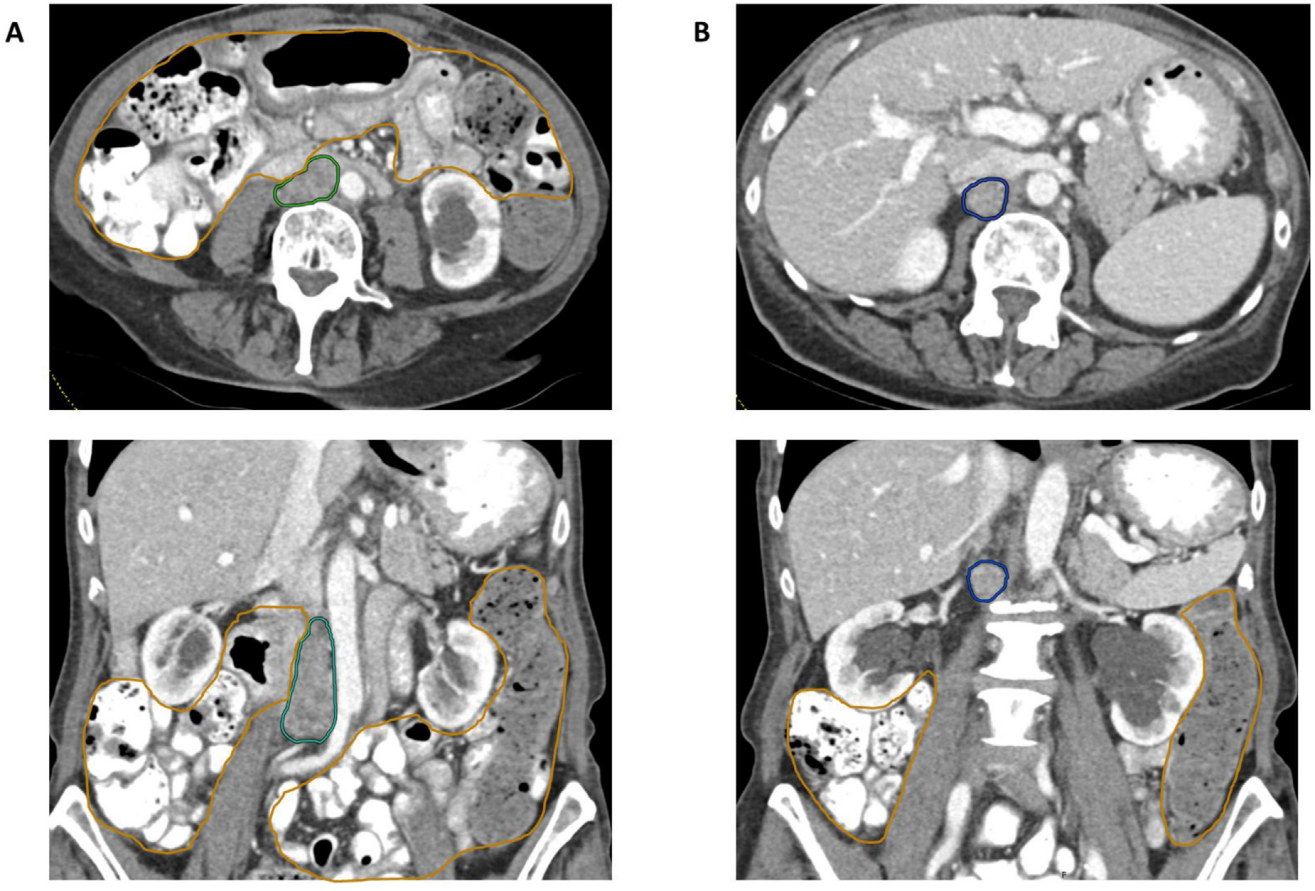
Computed tomography (CT) imaging of 2 pathologically enlarged abdominal lymph nodes concerning for disease progression and recurrence. (A) Axial and coronal intravenous (IV) and oral contrast-enhanced CT images of the abdomen and pelvis show an enlarging aortocaval lymph node (LN1), outlined in green, abutting the duodenum and measuring 18 × 19 × 35 mm, concerning for disease progression. (B) Axial and coronal contrast-enhanced CT images demonstrate an enlarged retroperitoneal lymph node (LN2), outlined in blue and measuring 13 × 15 × 18 mm, confirmed as recurrent cervical squamous cell carcinoma on biopsy. Adjacent bowel loops are outlined in orange.
